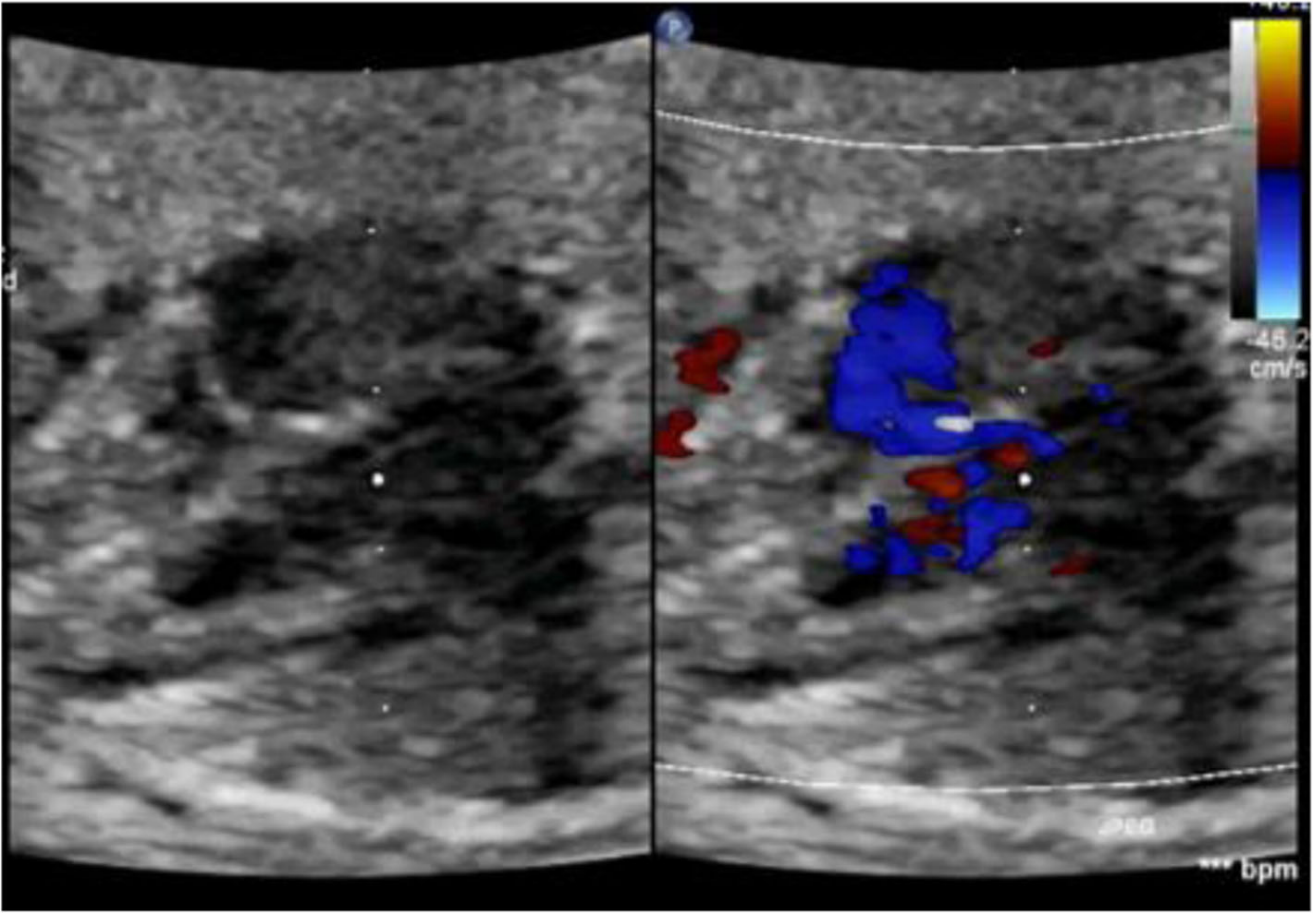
Four chamber view both by 2D and color Doppler imaging in a case 12-week gestation
